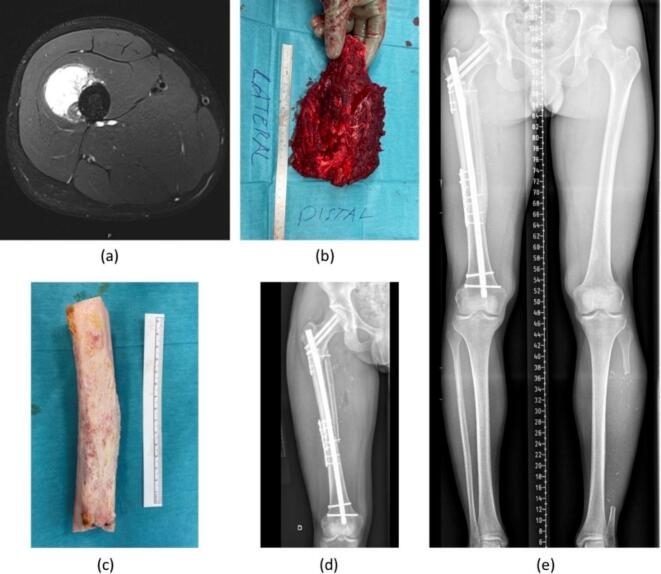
MRI scan showing periosteal osteosarcoma of the right femur in a 18-year-old male patient (a). En-bloc resection of the tumor bearing bone (b). After tissue removal, the bone was irradiated with 50 Gy using ECI (c). Re-implantation of the irradiated bone with orthopaedic fixation devices and a vascularized graft using the contralateral fibula (d). Bone union at the osteotomy sites 8 months after surgery (e).
